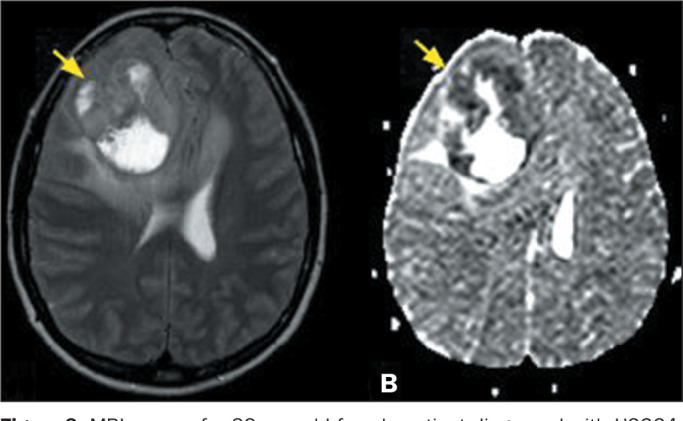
MRI scans of a 32-year-old female patient diagnosed with H3G34-mutant
